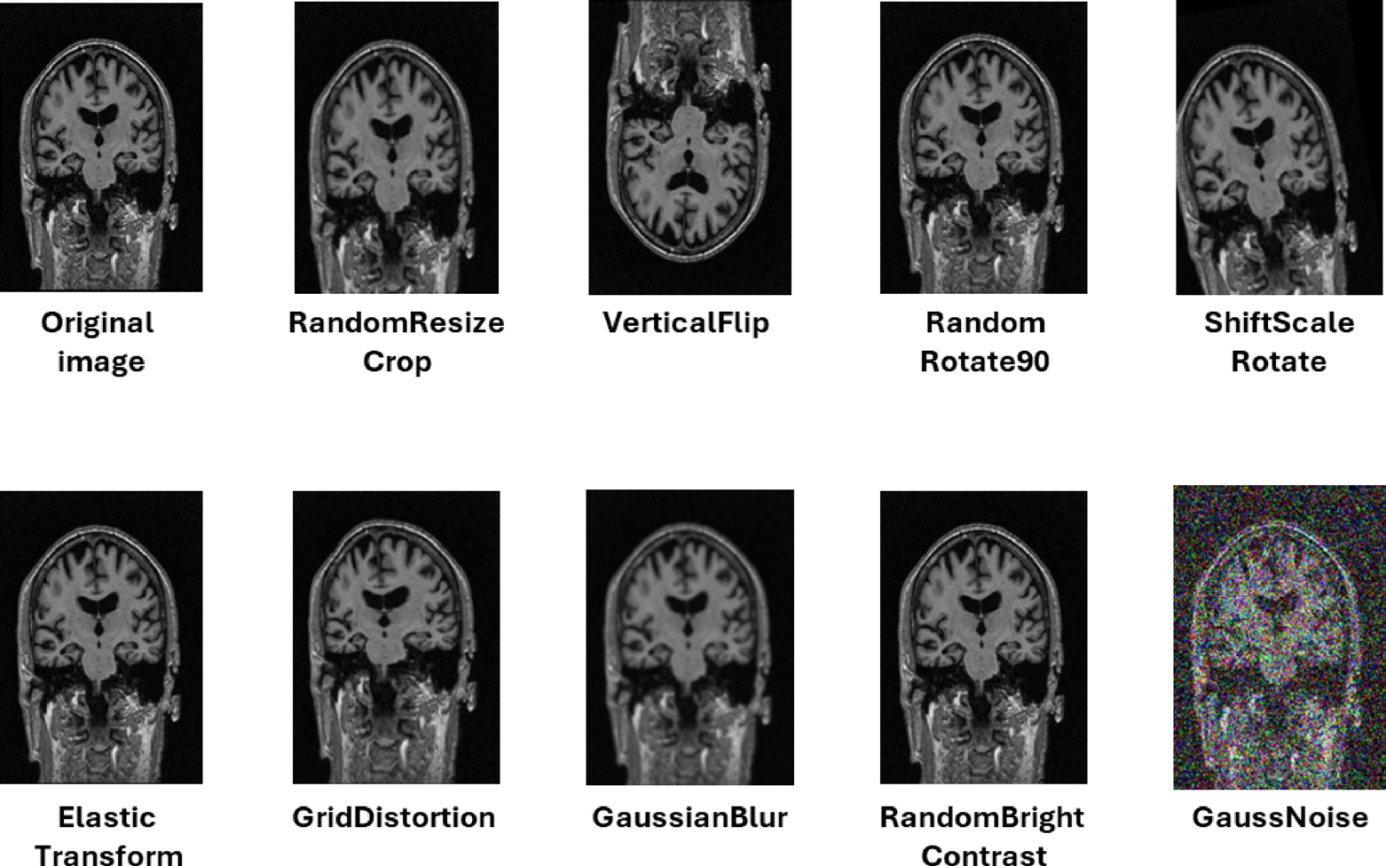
An example of data augmentation applied to the dataset with sample MRI images sourced from the Alzheimer’s disease neuroimaging initiative (ADNI) dataset.
